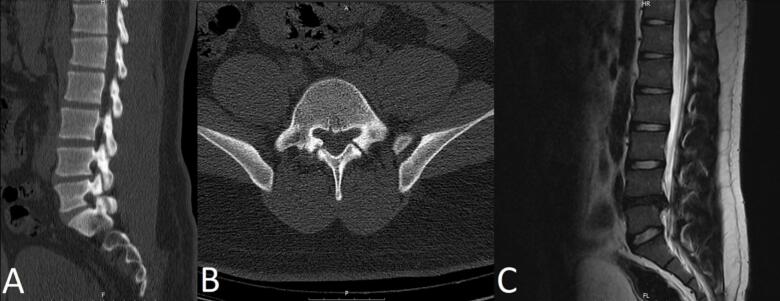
Preoperative imaging: Imaging of the lumbar spine showing bilateral spondylolysis at L5 (A, B) on preoperative CT scan and right-sided L4/L5 disc protrusion compressing the L5 nerve root (C) on MRI. No evidence of spondylolisthesis or endplate signal changes was observed.
